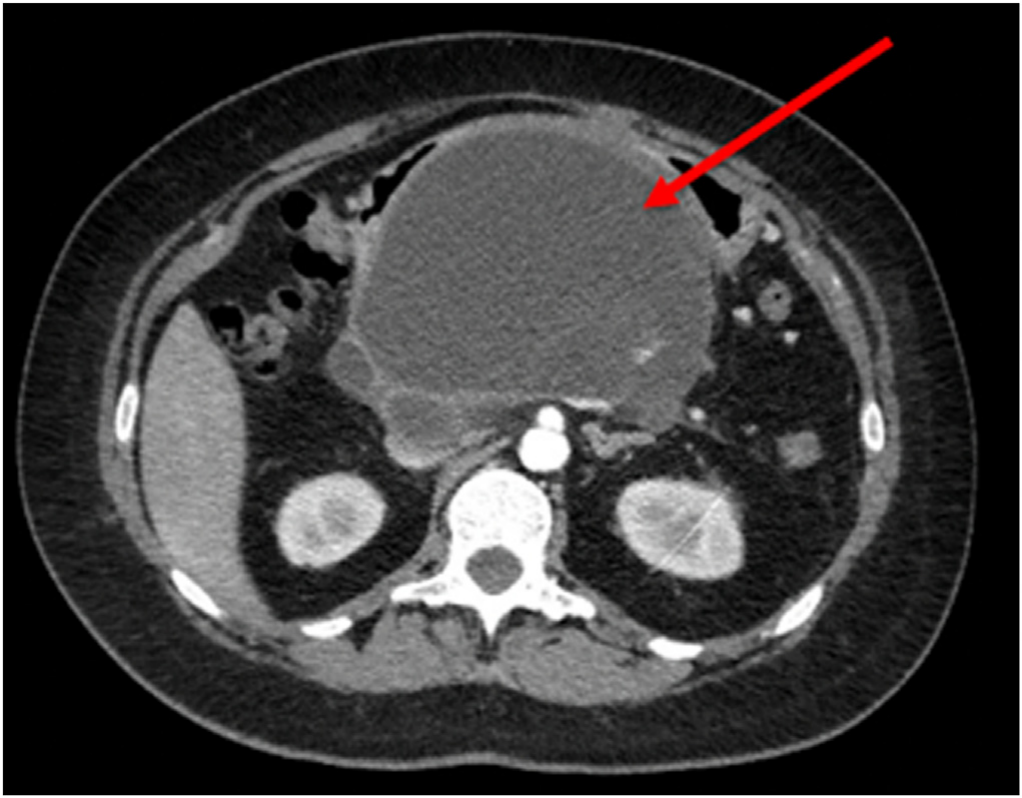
Computed tomography image demonstrating large pancreatic pseudocyst (*arrow*).
